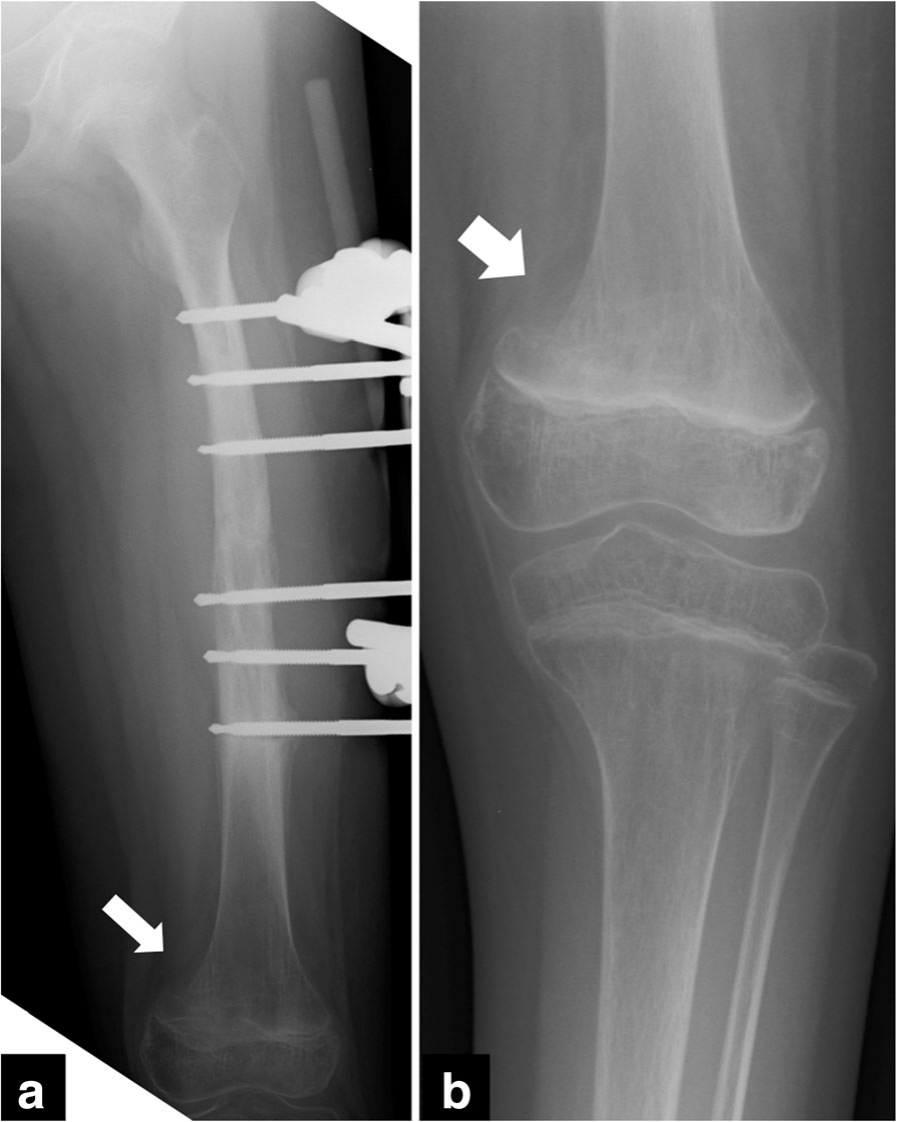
a A radiograph of the left femur presenting a supracondylar fracture at the distant site of the right femur. (arrow) (b) An enlarged image of the supracondylar fracture site
